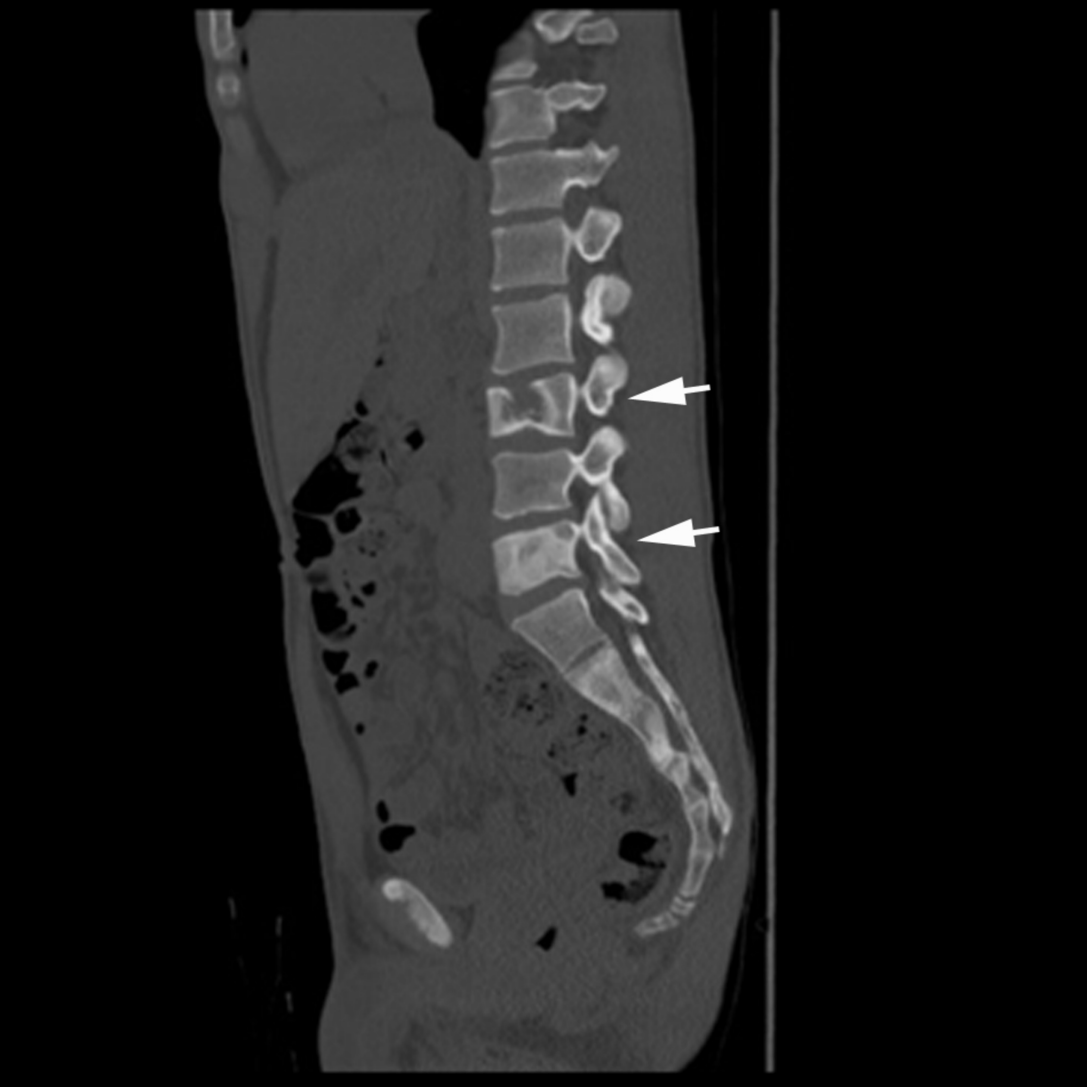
Admission computed tomography (CT) abdomen showed multiple osseous lesions with both osteosclerotic and osteolytic features as indicated by arrows.
